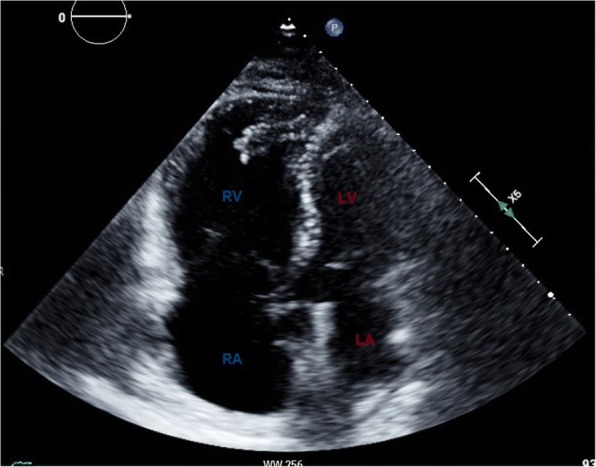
Four-chamber view on echocardiogram in a patient with CTEPH. Echo demonstrates RV and RA dilatation compared to the LV and LA (labeled)
